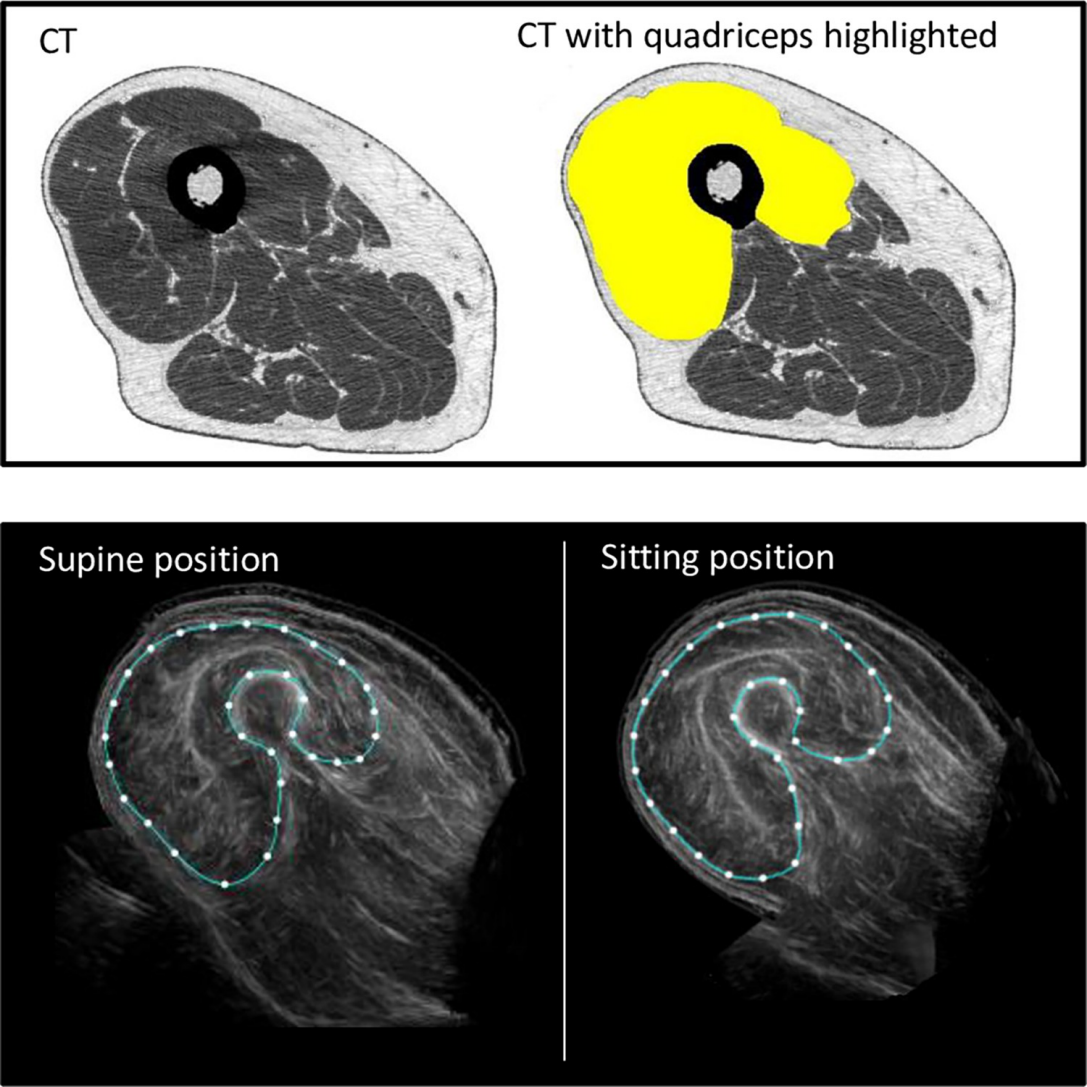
Three images of the same mid-thigh section using CT, supine ultrasound, and sitting ultrasound. Representative images of the same mid-thigh section using CT (upper), supine ultrasound (lower left), and sitting ultrasound young adults (lower right).
